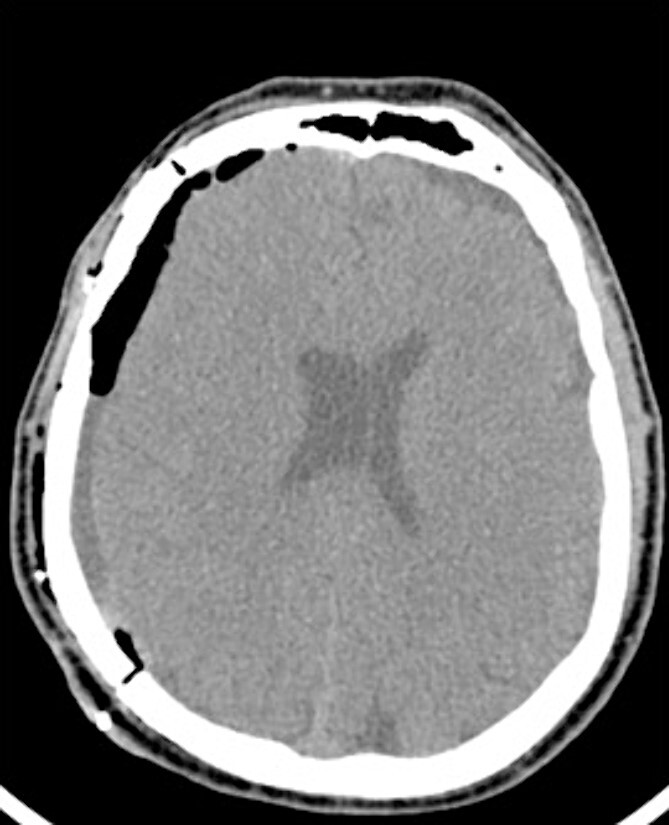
Postoperative CT imaging from Case 1.
